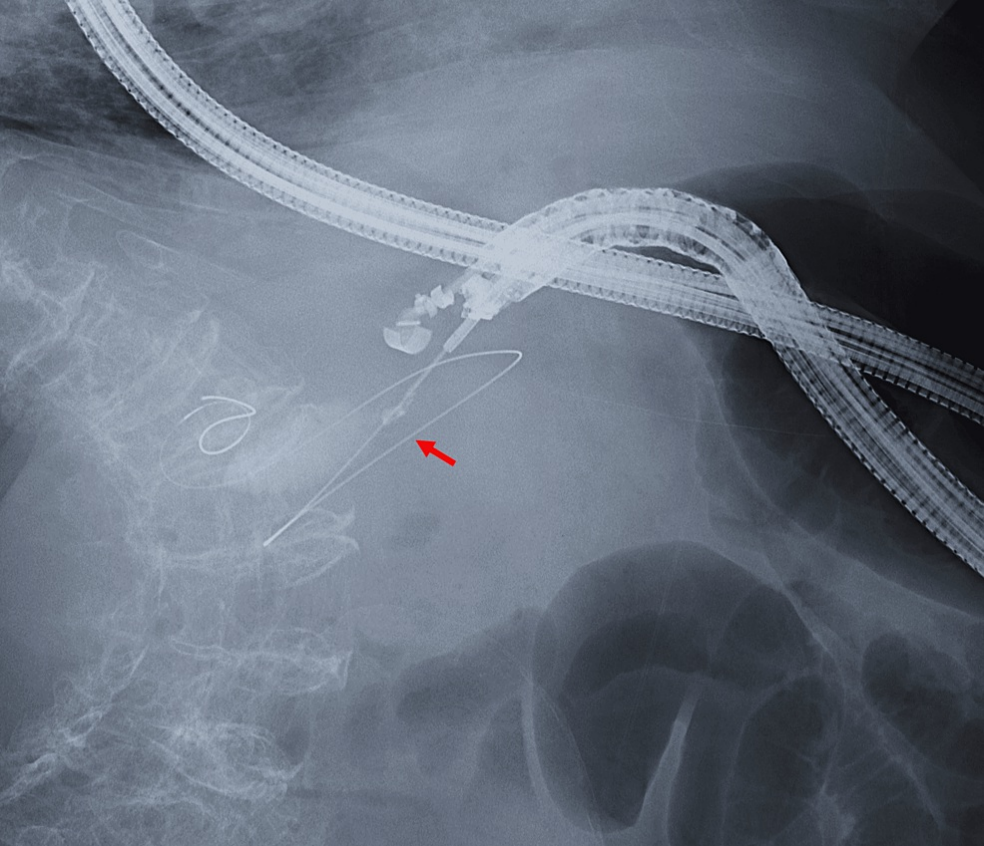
Fluoroscopy image during EUS-GBD A 0.025-inch guidewire (arrow) was introduced through the needle and coiled into the gallbladder. EUS-GBD: endoscopic ultrasound-guided gallbladder drainage
